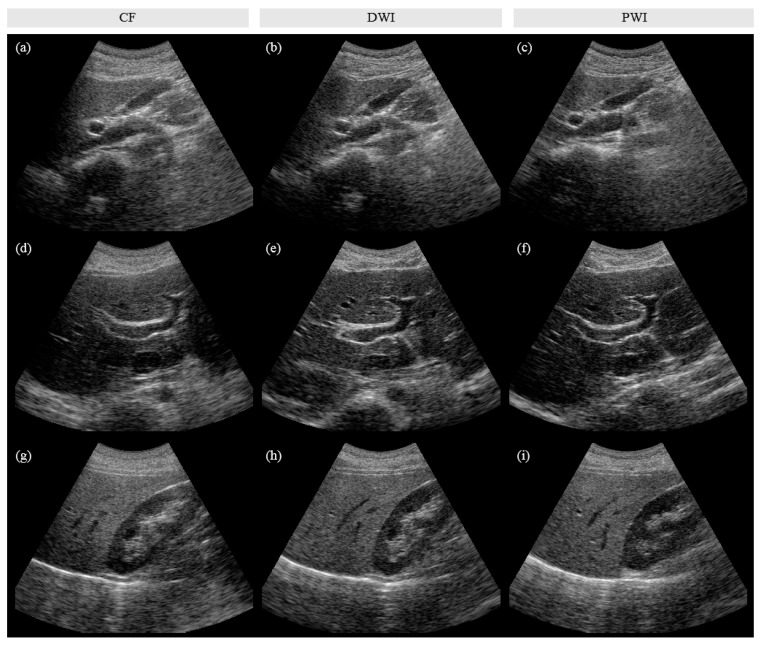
Representative in vivo images of (a–c) gallbladder, (d–f) liver, and (g–i) kidney, which were reconstructed by using CF (for (a, d, g)), DWI (for (b, e, h)), and PWI (for (c, f, i)). PWI showed better image quality with a 4-fold higher acquisition frame rate than CF and provided a comparable performance with a 2.9 times lower number of computations compared to DWI.
